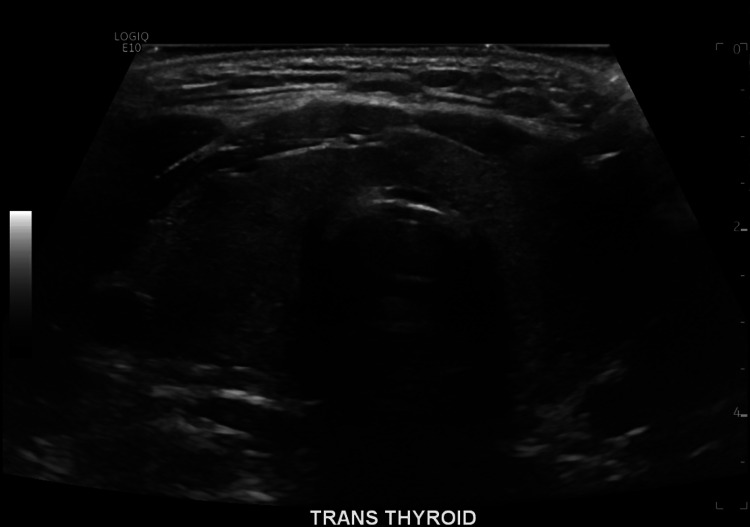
Ultrasound revealing an inhomogeneous, mildly enlarged thyroid
